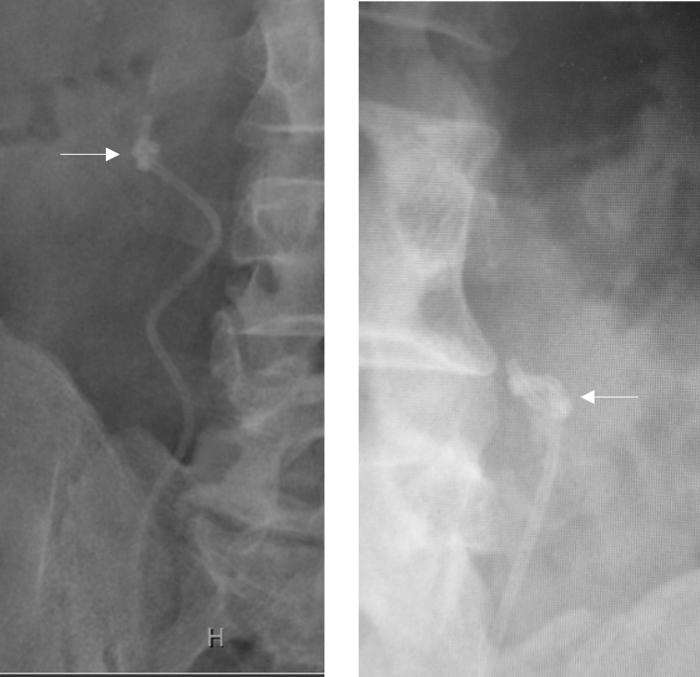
KUB X-ray showing a knot (indicated by *arrow*) in the upper curl of the ureteral stent.
